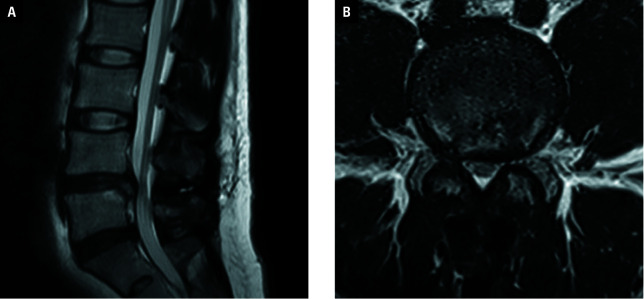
Postoperative magnetic resonance images of a 38-year-old man with huge lumbar disc herniation at the final follow-up; A – sagittal view showing satisfactory decompression and stable placement of the IntraSPINE device (arrow), with no exacerbation of intervertebral disc degeneration at the L4–L5 level; B – axial view showing satisfactory decompression and stable placement of IntraSPINE (arrow)
